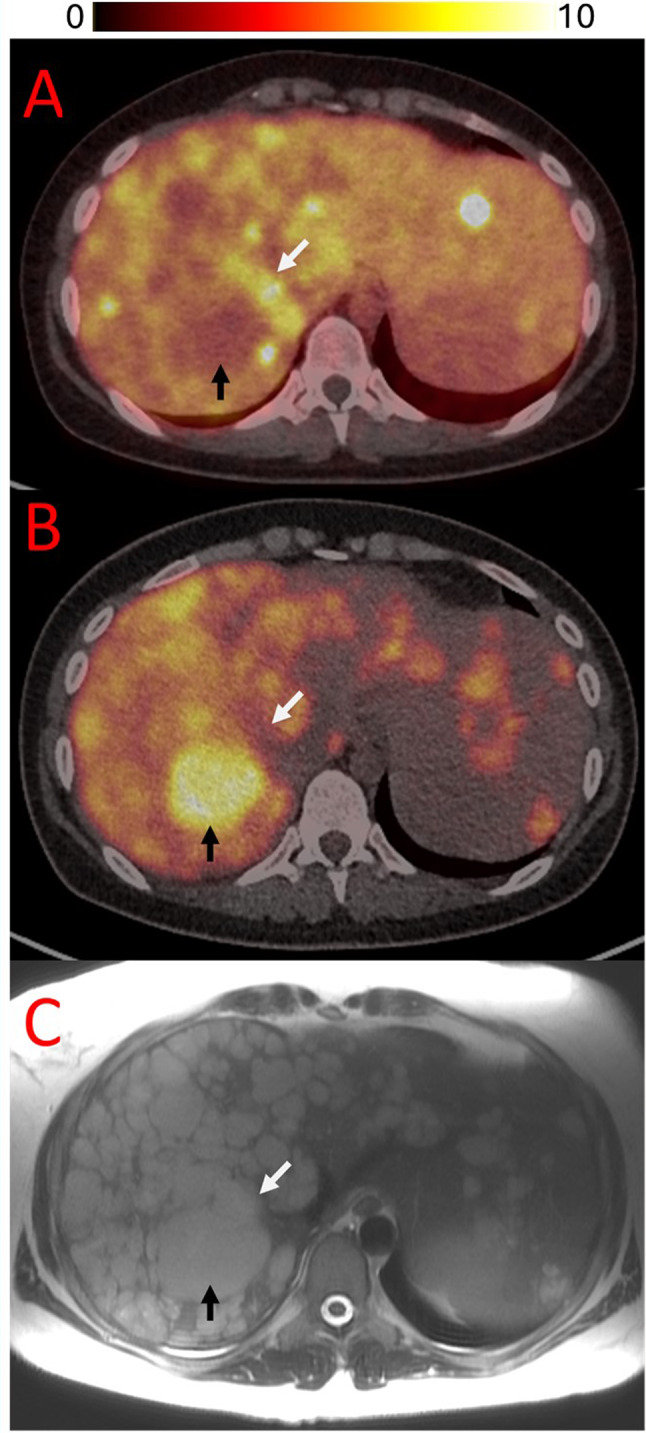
(A) Axial fused [^68^Ga] NeoB PET/CT demonstrating heterogenous uptake between liver metastases. (B) Axial fused [^18^F]-FDG PET/CT image and (C) correlative staging T2w MRI study at the same level. Multiple liver lesions are present, with differential uptake of NeoB and FDG. A lesion indicated in the right lobe demonstrates avid FDG uptake but photopaenia on NeoB PET (black arrow). Conversely, an adjacent lesion (white arrow) demonstrates uptake on NeoB PET without substantial FDG uptake
